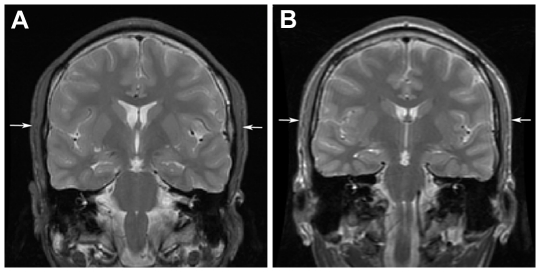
Attenuation of subcutaneous fat in Case 3. (A) Coronal T2-weighted image showing the patient has attenuation of scalp subcutaneous fat (arrows). (B) Normal appearance in a patient with epilepsy without 17q12 deletion (arrows). Subcutaneous scalp fat was present on cranial MRI in 150 patients with temporal lobe epilepsy who did not carry this deletion.
